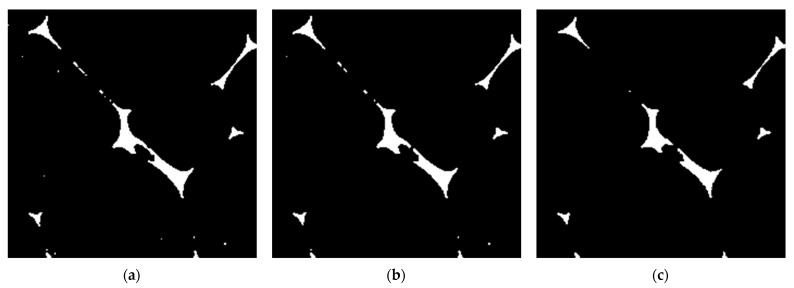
Part of binarized images in Figure 7a scanned by MCT225 Metrology CT (enlarged image of an area of approximately 0.90 × 0.90 mm^2^) with thresholds of (a) 0.499, (b) 0.500, and (c) 0.520.
